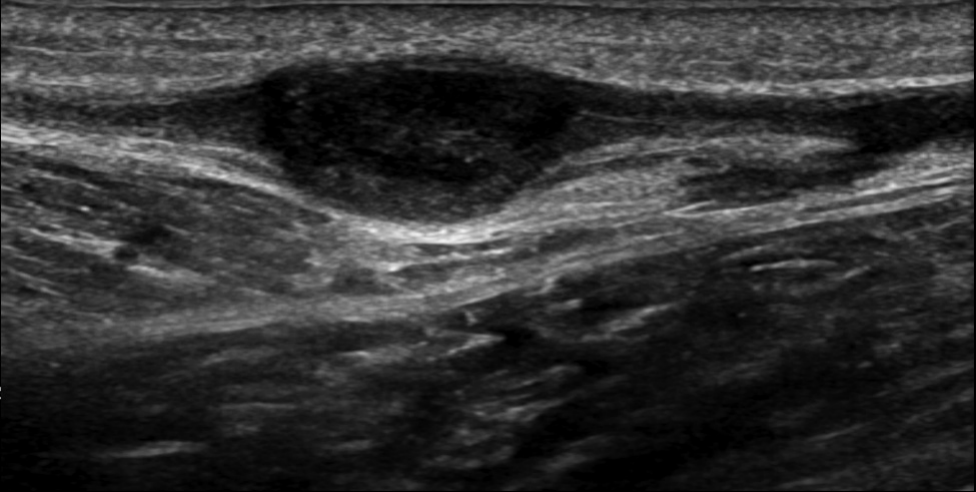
Ultrasonography findings of intraneural hematoma on the right calf.
